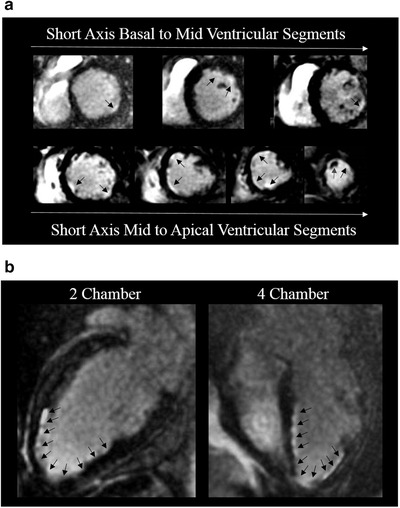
Patient 1 cardiac MRI. A, Serial short axis delayed contrast enhancement images from base to apex. Black arrows demonstrate areas of late gadolinium enhancement. Purple arrow demonstrates an apical thrombus. B, Left panel: Four chamber delayed contrast enhancement image; right panel: Two chamber delayed contrast enhancement image. Black arrows demonstrate areas of late gadolinium enhancement
